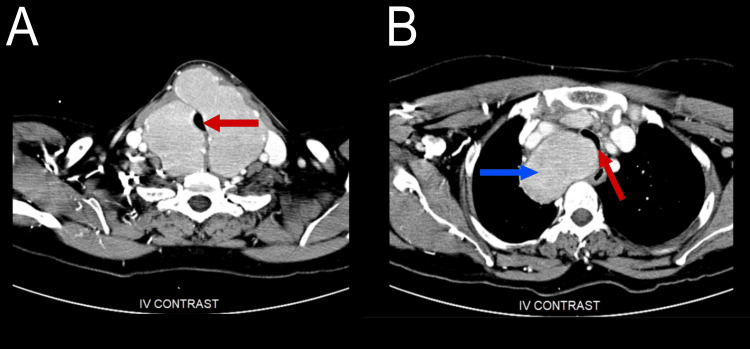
CECT images of a 47-year-old patiemt with multinodular goiter. Image A shows the enlarged thyroid gland compressing the trachea, causing luminal narrowing. Image B shows retrosternal extension of the enlarged thyroid gland with tracheal compression. The red arrow indicates tracheal compression and the blue arrow indicates the retrosternal extension of the enlarged thyroid gland. CECT: contrast-enhanced computed tomography.
